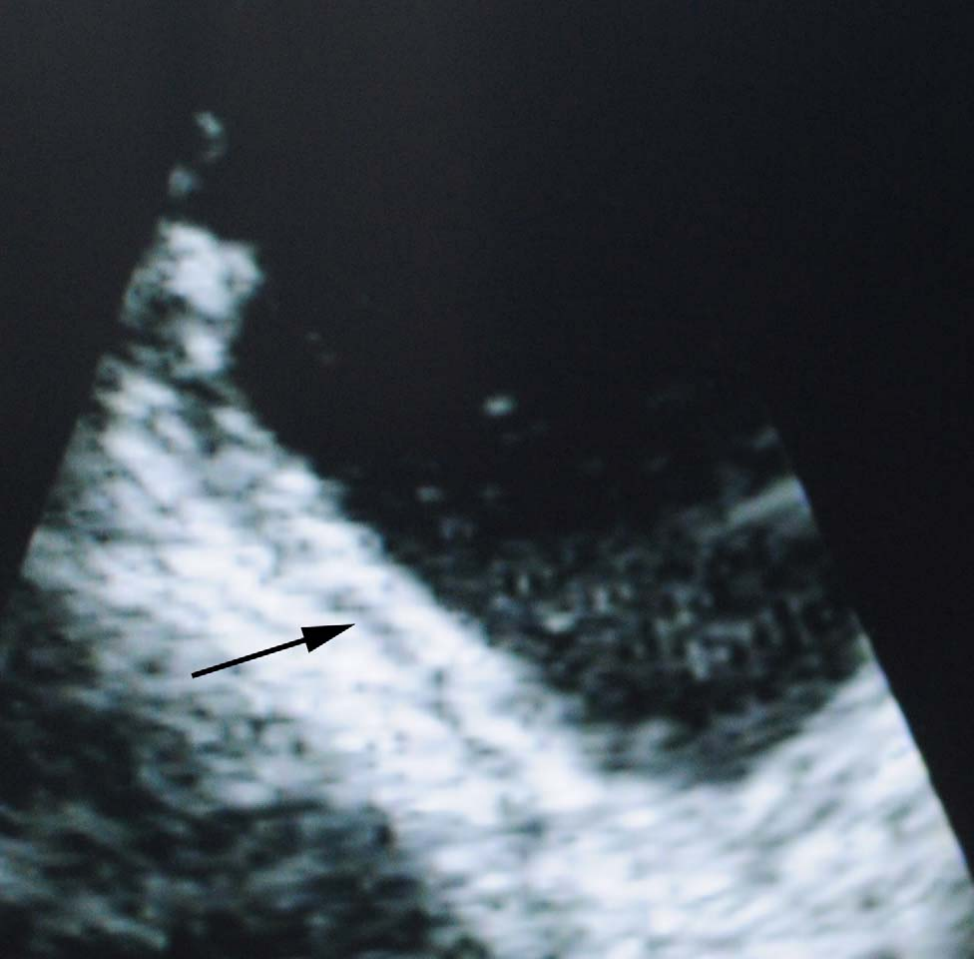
The normal muscle layer of bladder wall in the ultrasonic image.
